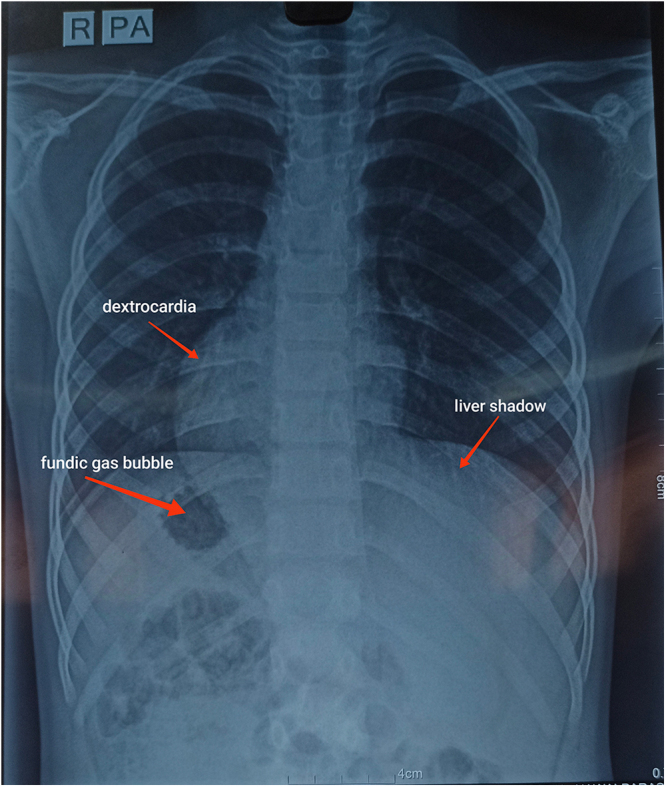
Chest X-ray PA view showing dextrocardia with aortic arch curving toward right, stomach fundic gas bubble under the right hemidiaphragm, liver shadow under left hemidiaphragm.
